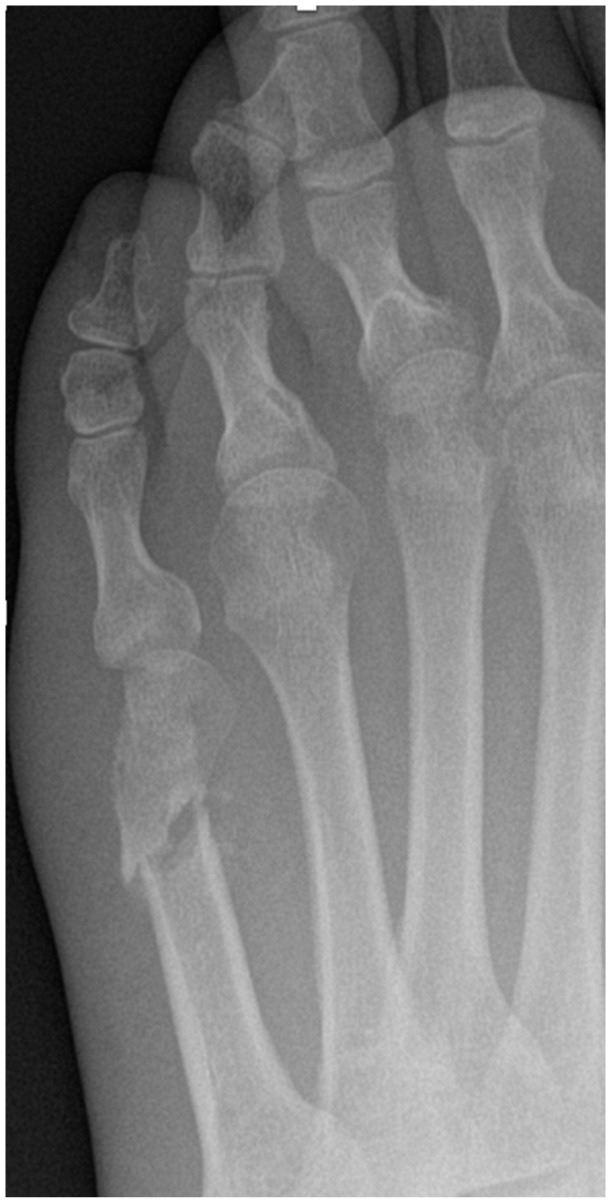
Radiograph showing complete osteotomy with disrupted lateral cortical hinge.
